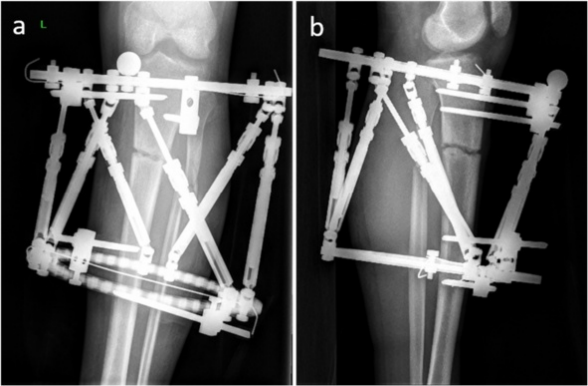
a, b The postoperative third-day radiograph of the fracture after correction of the deformity
